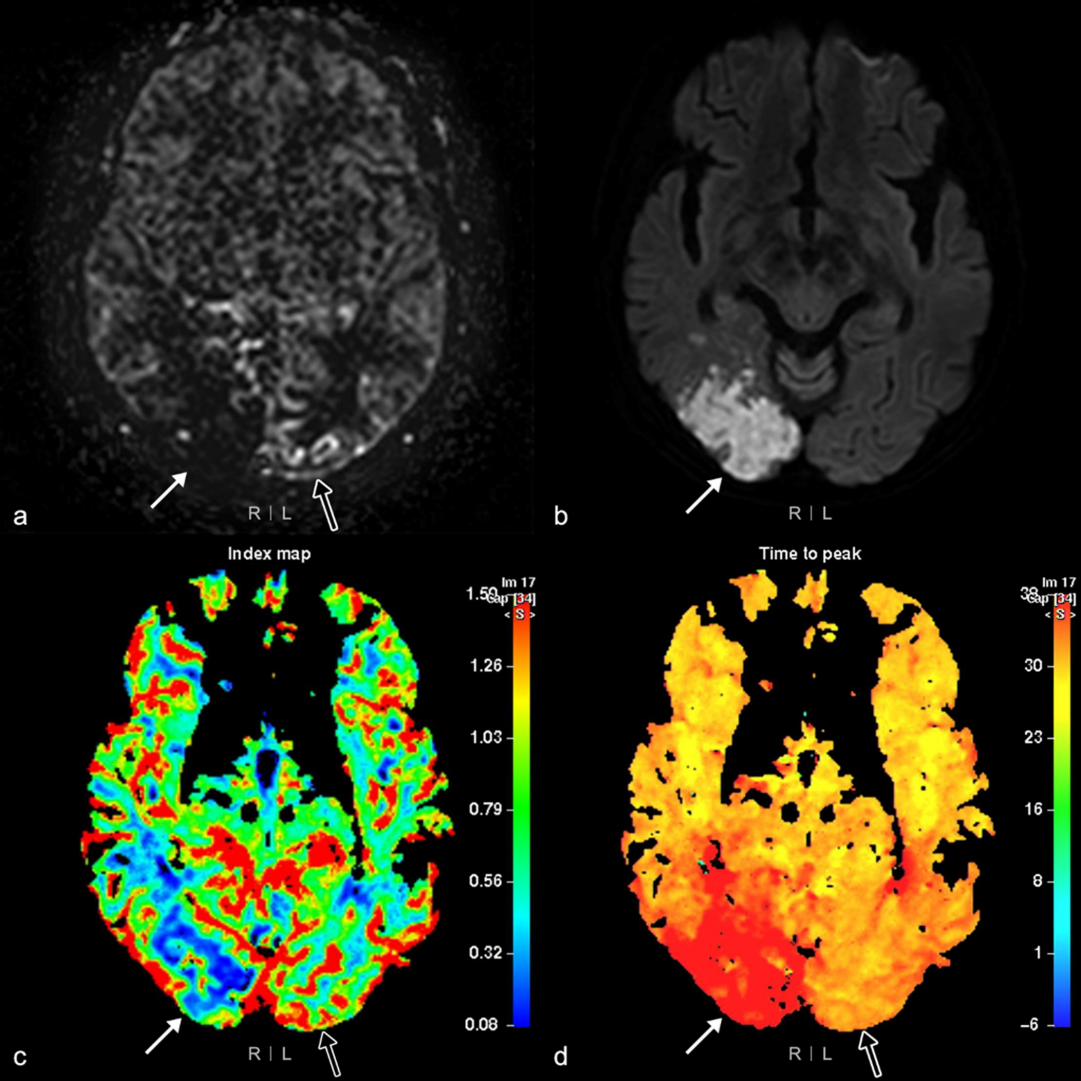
Arterial spin labeling (ASL) rank II and IV perfusion abnormalities. MRI of the brain of a 78-year-old woman with a left visual field defect. (a) ASL imaging shows a rank IV perfusion abnormality; positive parenchymal perfusion deficits (PPD) in the right occipital area (arrow); (b) Diffusion weighted imaging (b = 1,000) shows a high signal intensity (arrow); (c) cerebral blood flow (CBF) and (d) time to peak (TTP) maps show matched perfusion defects (arrow) in the same area. (a) A rank II perfusion abnormality is visualized as positive hyperintense vessel signals without PPD (open arrow) using ASL imaging, and a normal (open arrow) is seen in the left occipital area on (c) CBF and (d) TTP maps.
